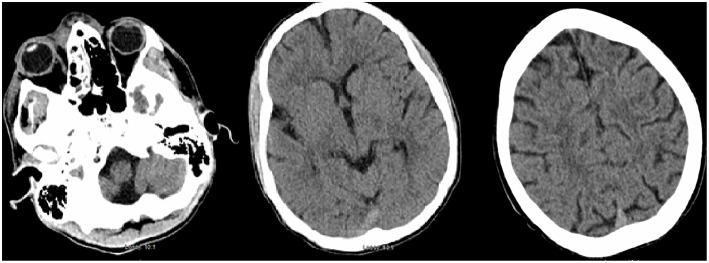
Initial CT head demonstrating periorbital edema and inflammation in the ethmoid and maxillary sinuses. There is no evidence of acute intracranial abnormality.
